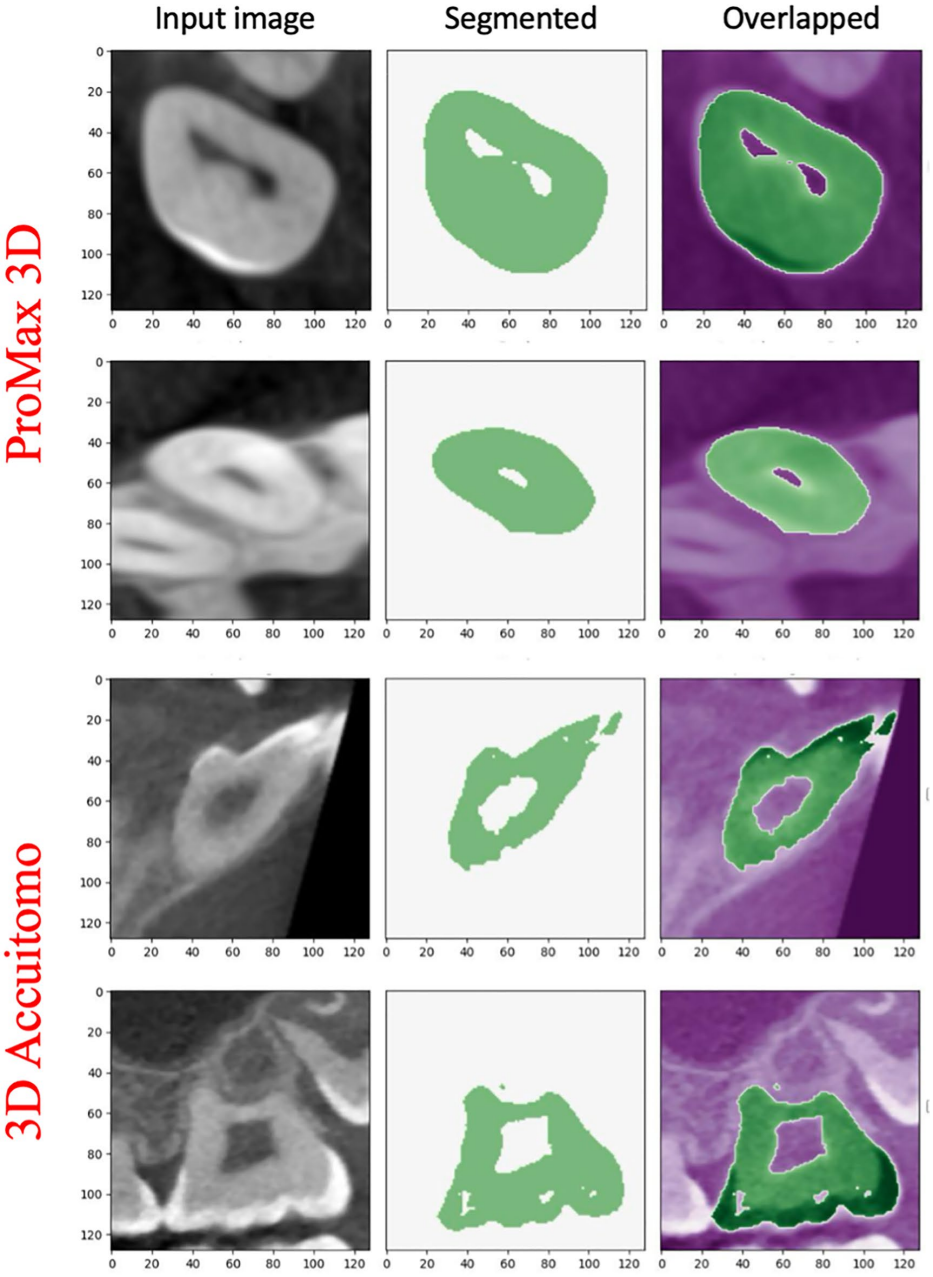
Segmentation by DL model developed with virtual CBCT images. The representative result in the input CBCT acquired by the ProMax 3D scanner and by the 3D Accuitiomo are shown. “True” means manual segmentation, whereas “Pred” means DL segmentation. The difference images between “True” and “Pred” are depicted in the last column.
